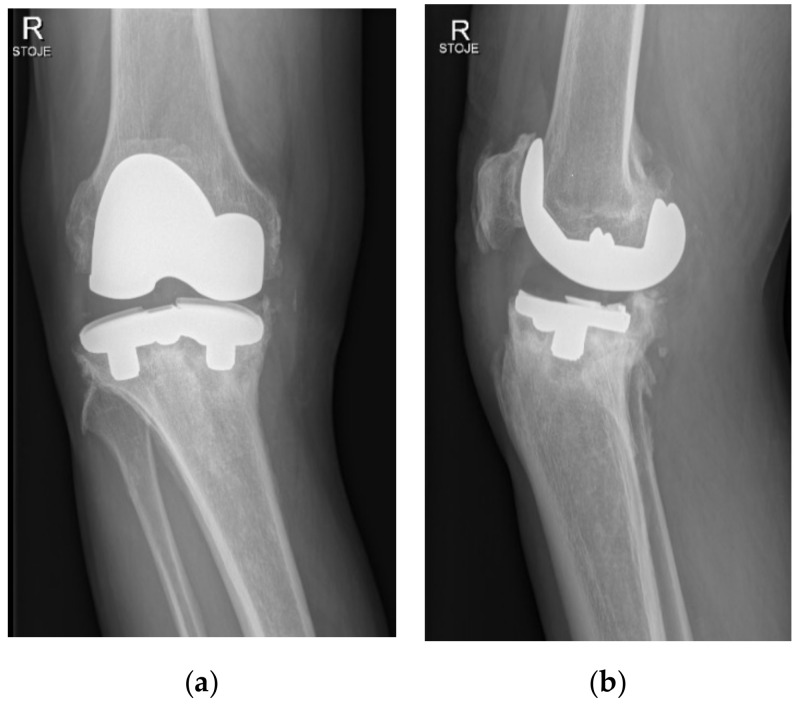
Anteroposterior (a) and lateral (b) standing radiographs 3.5 years after index operation depicting fracture and displacement of the medial part of the tibial tray.
